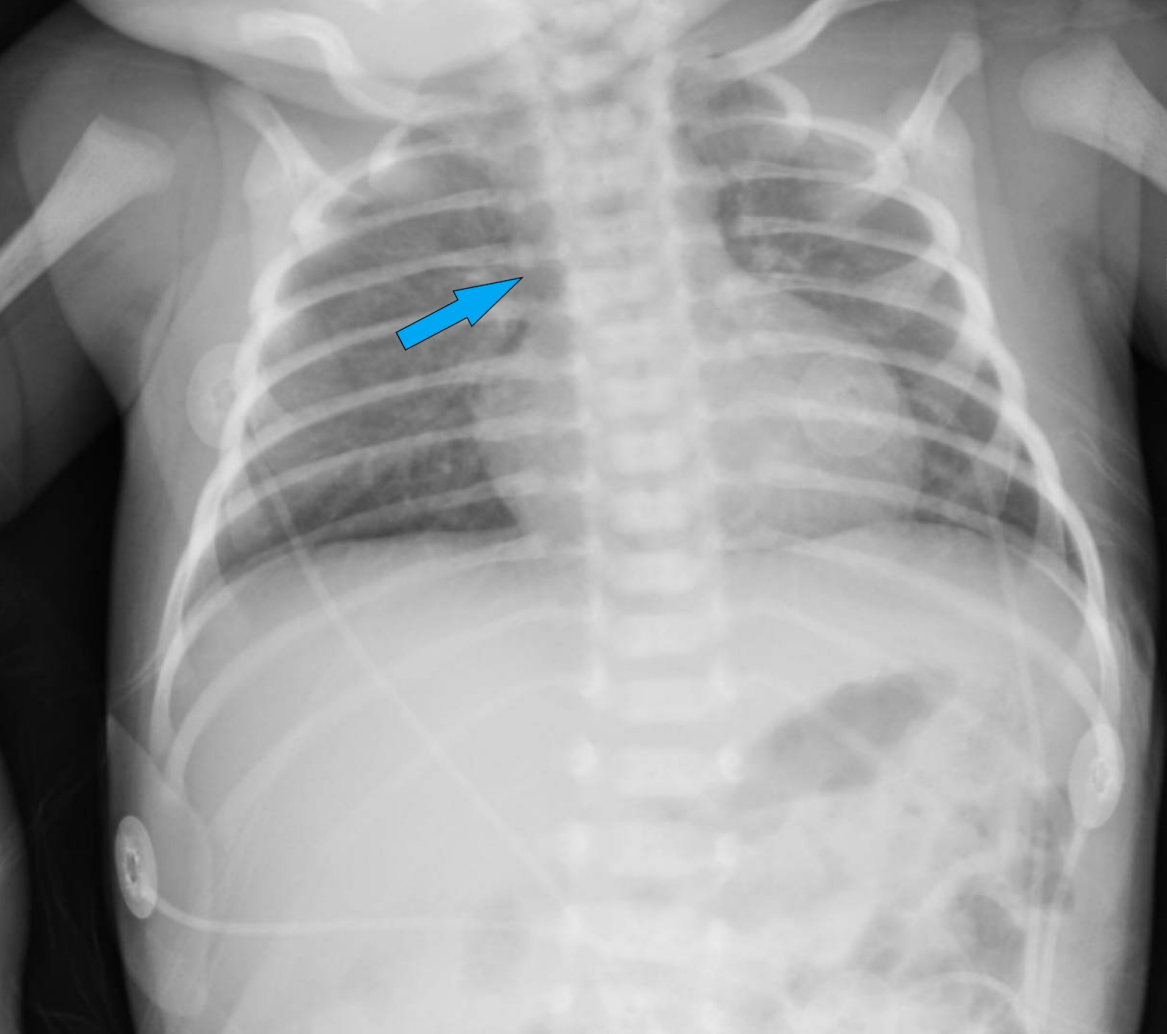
Chest X-ray demonstrating absence of the thymic shadow (arrow).
